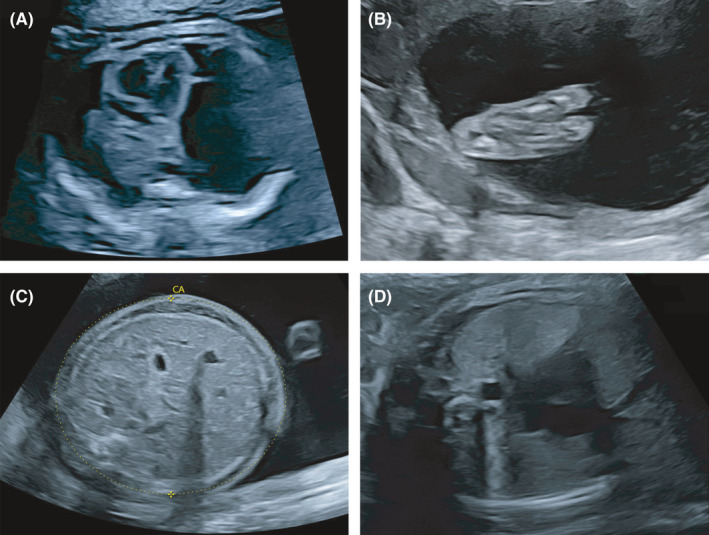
A, Ultrasound examination at 21 5/7 gestational weeks showing axial view of the fetal chest with bilateral hydrothorax and (B) ectrodactyly of the left foot. C, Ultrasound examination at 26 5/7 gestational weeks showing axial view of the fetal abdomen with periabdominal edema, no ascites and an abdominal circumference (CA) of 271.0 mm (>99th percentile). D, Ultrasound examination at 35 2/7 gestational weeks showing axial view of the fetal chest with hydrothorax resolution
